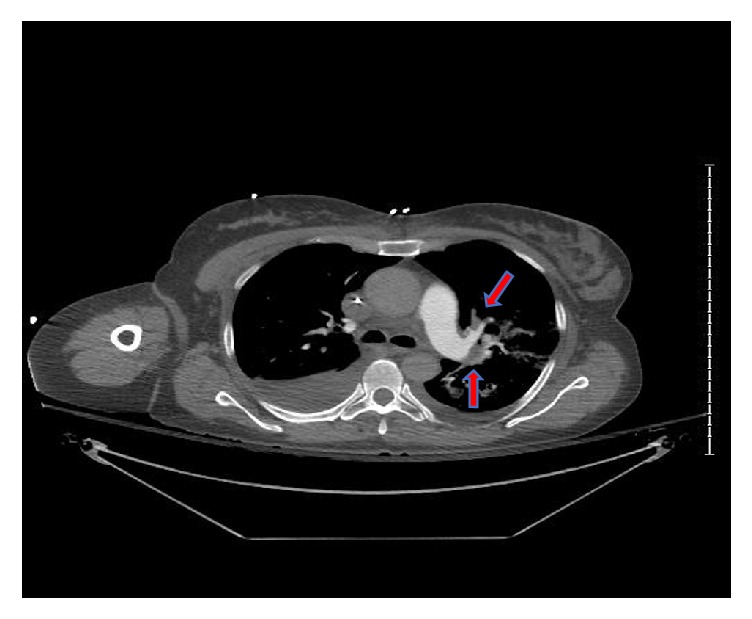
CT angiogram showing pulmonary embolism (arrow).
